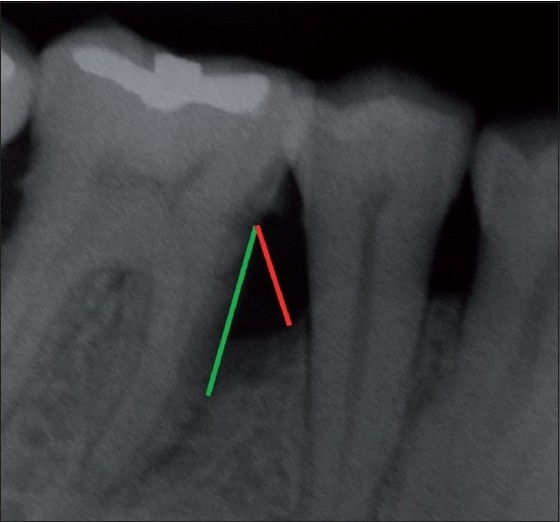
Linear radiographic interpretation with Computer Image analysis software. (Green line indicates CEJ to base of defect, red line indicates CEJ to alveolar bone crest)
